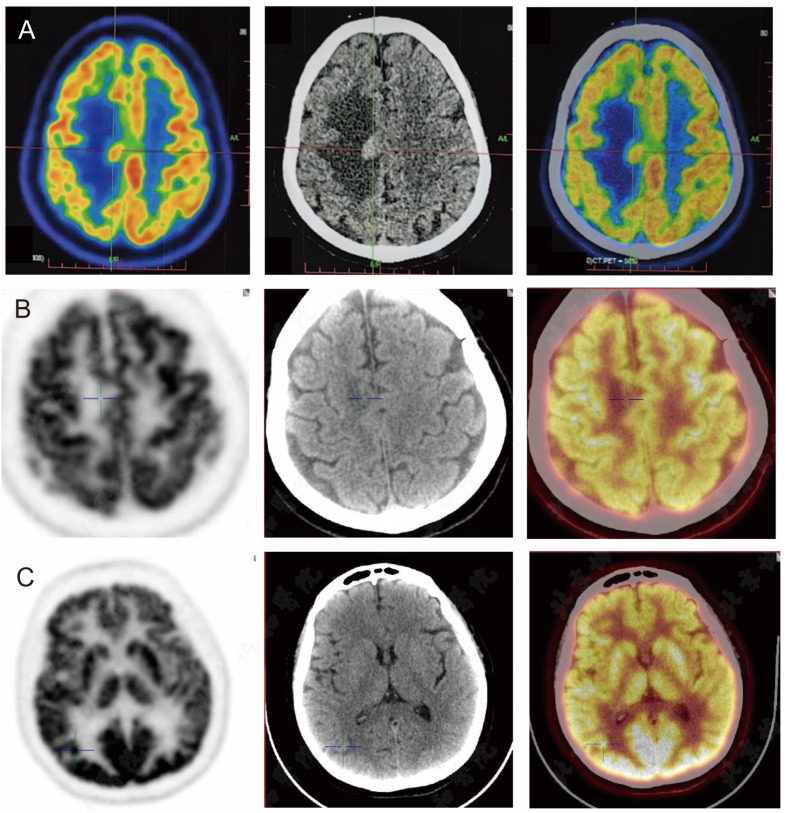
PET/CT images of the patient’s brain during the diagnosis and treatment. (A) 22 April: a hypermetabolic focus with peripheral edema in the right centrum semiovale (SUVmax 14.3); (B) 8 July: nodules of centrum semiovale in the right, metabolically lower than the cortex; (C) 8 July: nodules of increased metabolism in the right occipital lobe (about 0.7 cm, SUVmax17.4).
